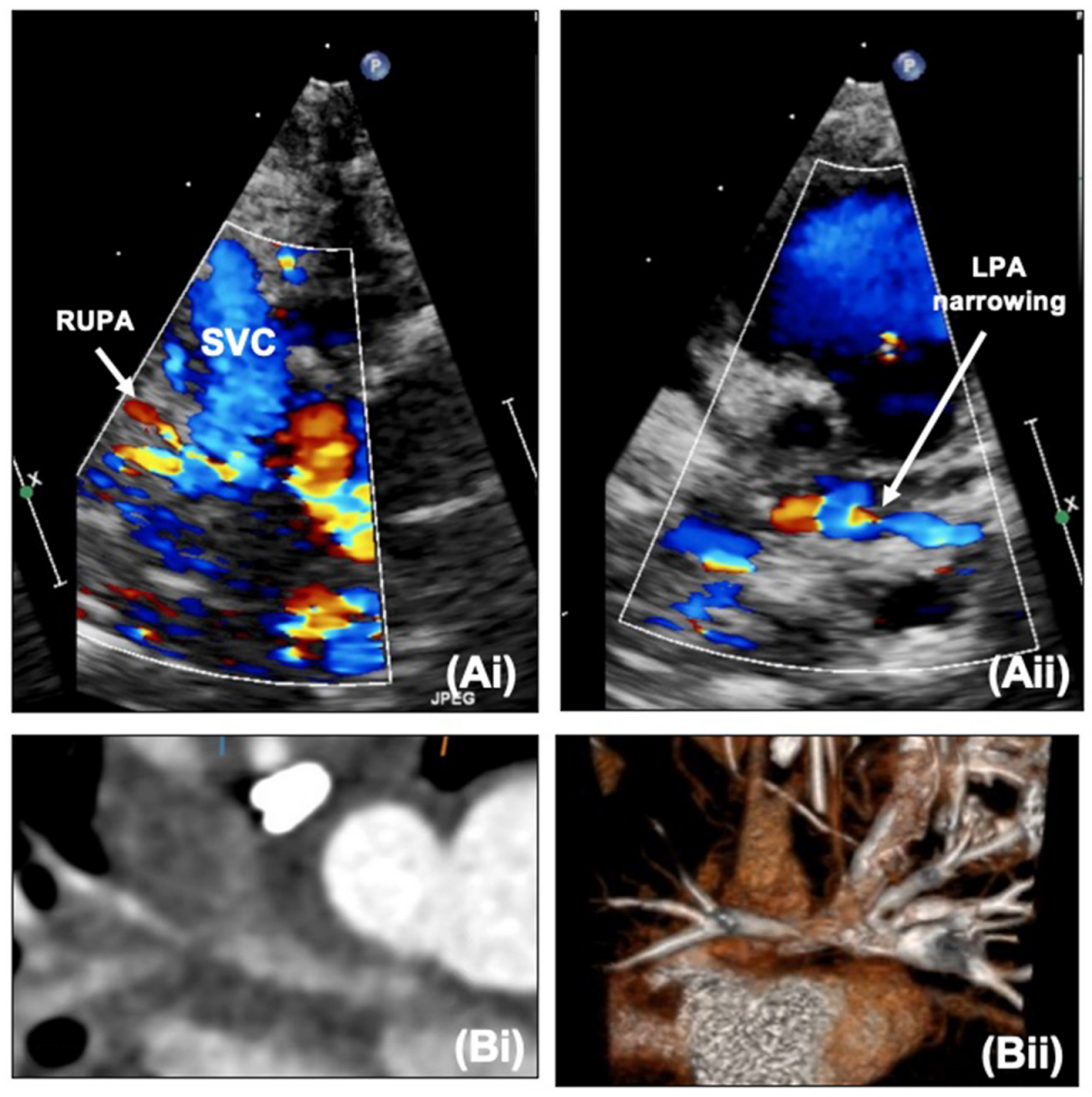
(A,i) Right upper pulmonary artery narrowing with Glenn on echocardiography. (A,ii) Proximal left pulmonary artery narrowing – site of previous pulmonary artery band. (B,i) Narrowing of the right pulmonary artery at the bifurcation. (B,ii) Proximal left pulmonary artery narrowing on CT. LPA, Left pulmonary artery; RUPA, right upper pulmonary artery; SVC, superior caval vein.
